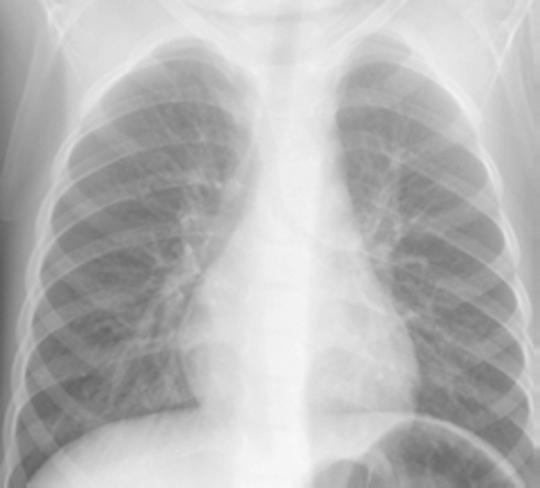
Chest radiograph showing interstitial infiltrates
